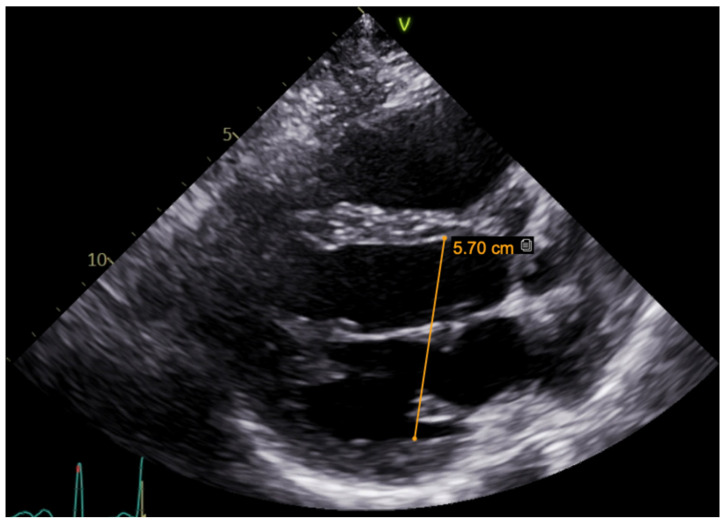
TTE parasternal long-axis view before treatment (moderately dilated left ventricle, LVdd 57 mm).
